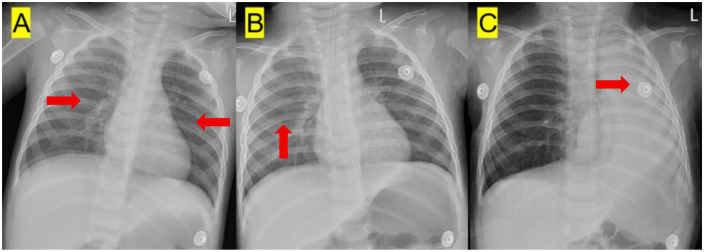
(A) Chest x-ray obtained at 23:42 (on admission) showing increased bronchovascular markings. (B) Chest x-ray obtained at 01:49 (2 h post-admission) showing increased radiopacity of the right lung. (C) Chest x-ray obtained at 06:24 (6 h and 40 min post-admission) showing complete left lung consolidation with collapse.
